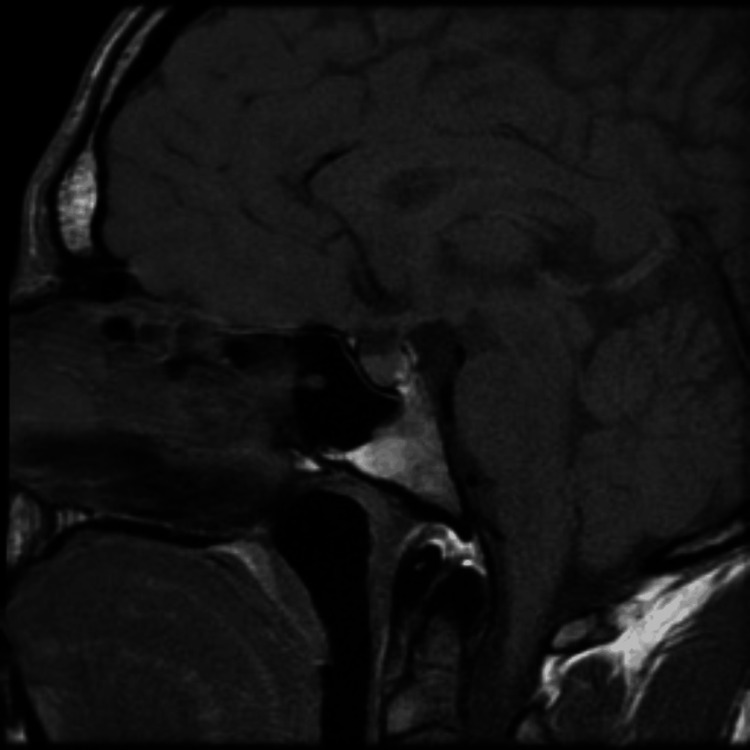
Midline sagittal T1-weighted sellar MRI. Pituitary and sellar area without significant changes. MRI: magnetic resonance imaging
